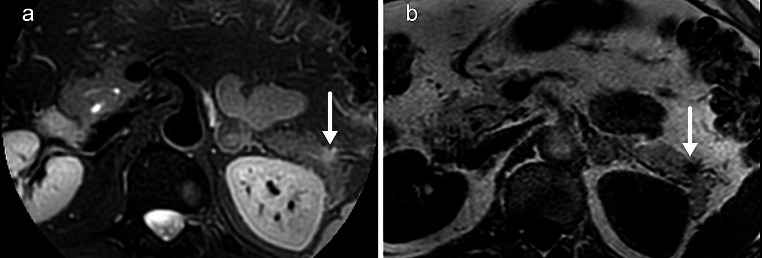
Small hyperintense lesion in the pancreatic tail on fat-suppressed T2-weighted turbo-spin echo sequence with radial k-space acquisition (a). B shows the lesion on T1 with presaturation pulse for comparison
